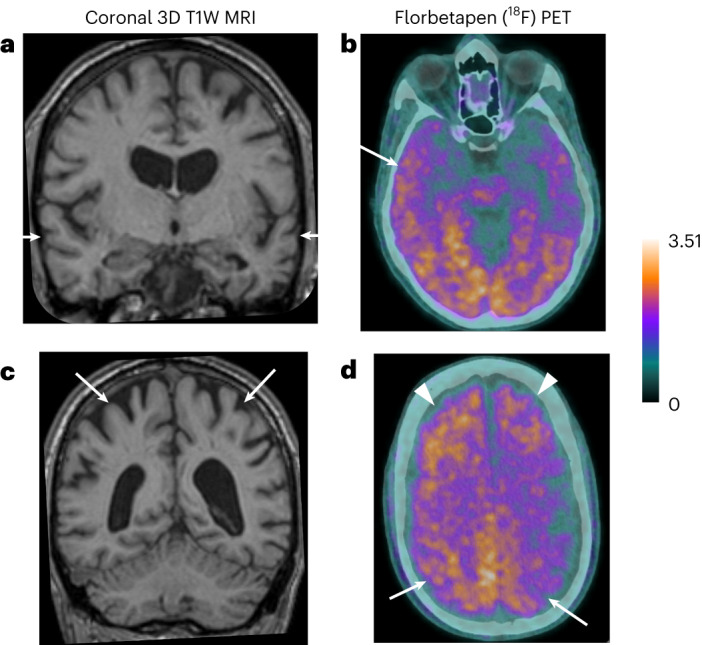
Magnetic resonance and amyloid-PET (^18^F-Florbetapen) images—case 3. a, High-resolution three-dimensional (3D) T1-weighted (T1W) magnetic resonance (MR) coronal image through the temporal lobes demonstrates volume loss within the temporal lobes bilaterally (arrows) and also marked central atrophy. b, Axial PET images demonstrate diffuse increased tracer uptake in the cortex and subcortical white matter, increased in the right temporal lobe compared to the left. c, High-resolution MR (3D T1W) coronal image through superior parietal lobules bilaterally demonstrates marked volume loss (arrows). d, Axial PET images demonstrate marked tracer uptake within the superior parietal lobules bilaterally (arrows) in addition to increased uptake in the bilateral frontal lobes (arrowheads).
